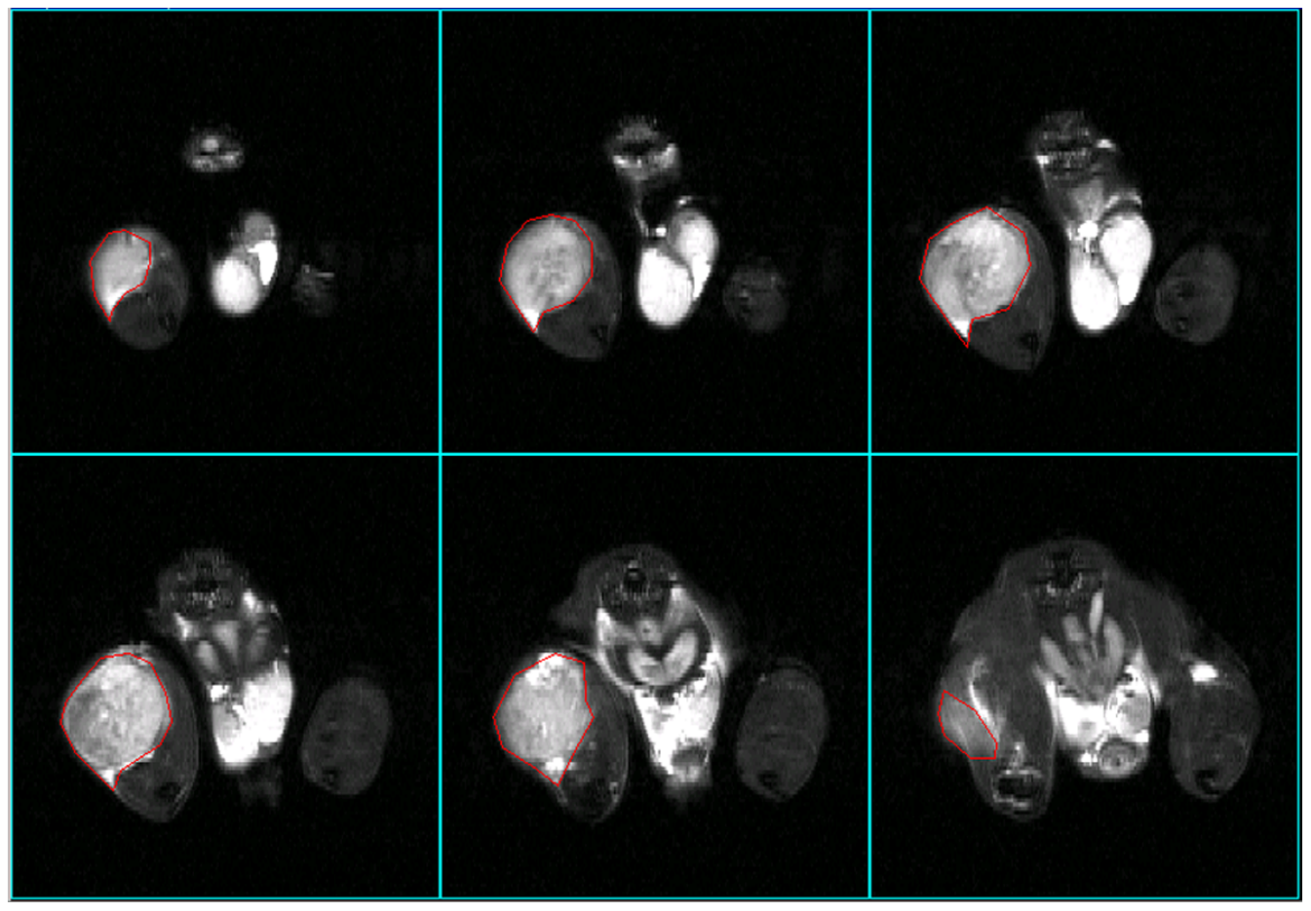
MR imaging of primary tumour with measurement of tumour volume. Measurement of a VH-64 Ewing sarcoma of the injected femur with a large extra-osseous tumour component. The regions of interest (within red markings) were measured in sequential 2 mm thick slices that showed tumour, and by adding the volumes of individual slices the total tumour volume was calculated. In the depicted case the estimated total volume was 492 mm^3^ (tumour in 6 slices; region of interest per slice: 22 mm^2^, 42 mm^2^, 57 mm^2^, 59 mm^2^, 52 mm^2^, 14 mm^2^; total area = 246 mm^2^; slice thickness = 2 mm; estimated total volume = 492 mm^3^).
